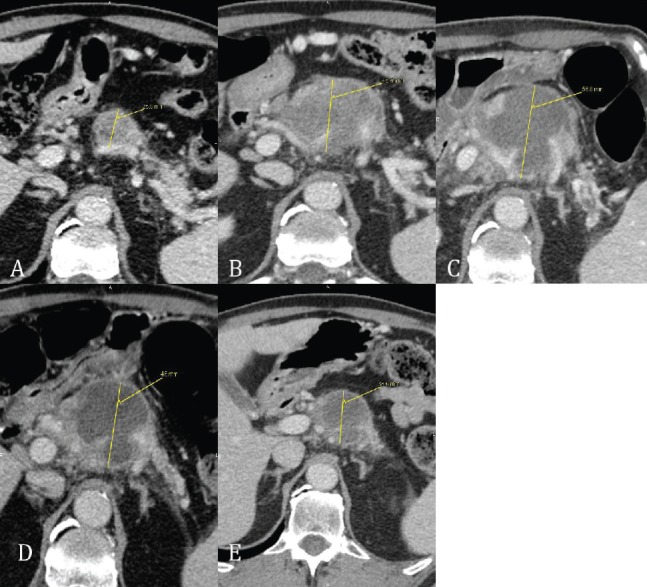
(A) Baseline CT scan revealed an inhomogeneous hypodense lesion in the head of pancreas; (B) after chemotherapy with gemcitabine plus cisplatin; (C) within 24 hours after HIFU pancreatic lesion shows a lump of necrotic tissue (between thrid and fourth FOLFIRI cycle); (D) CT scan at six weeks after HIFU; (E) 16 weeks after HIFU.
